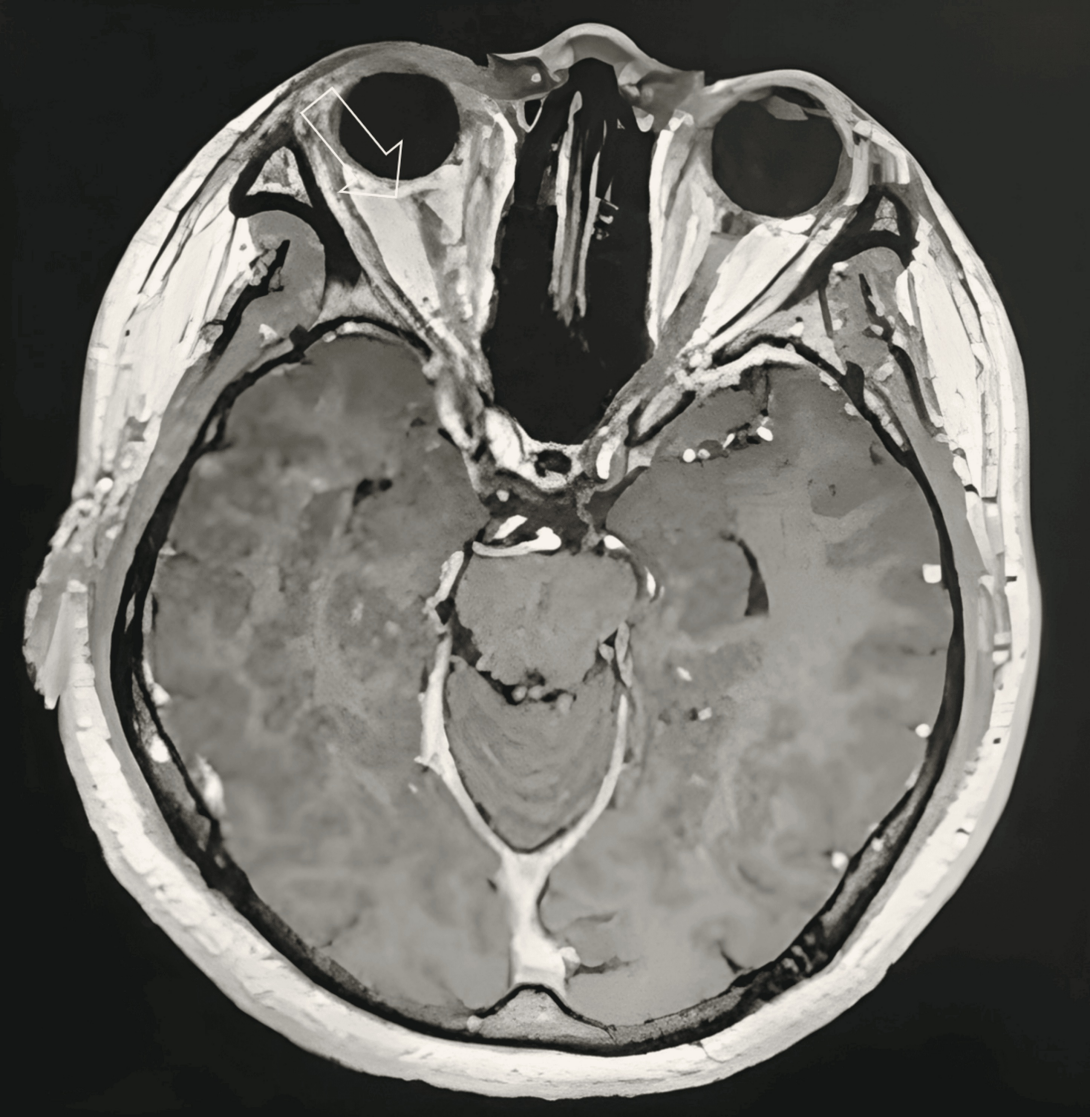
Axial cut MRI of the brain and orbit showing mild heterogenous hyperintensity along the right optic nerve (arrow) with no evidence of central nervous lesion suggestive of demyelinating disease MRI: magnetic resonance imaging
